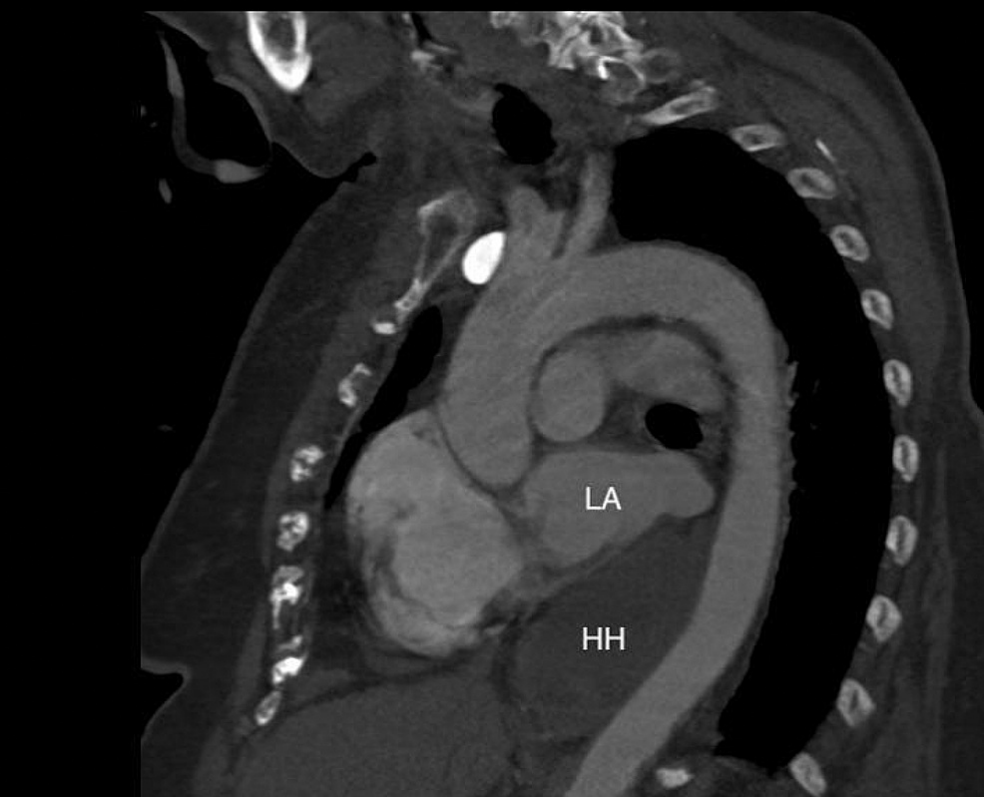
Sagittal view of CT chest with compression of the posterior left atrium by a giant hiatal hernia. LA: left atrium; HH: hiatal hernia
